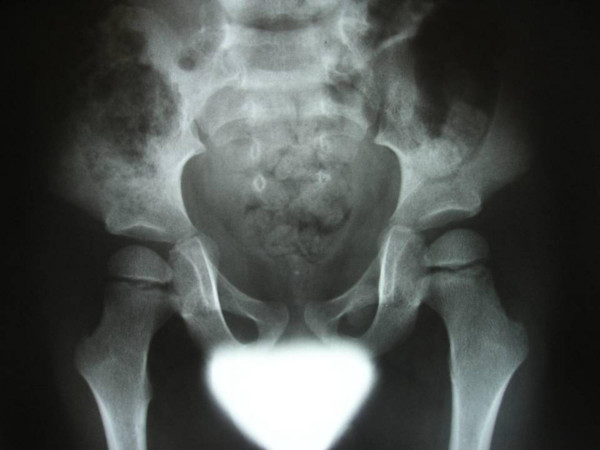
Anteroposterior lower pelvis and long bones radiograph showed Coxa valga, long femoral neck and inward curvation of the femoral shafts associated with widened metaphyses of the inferior femora.
